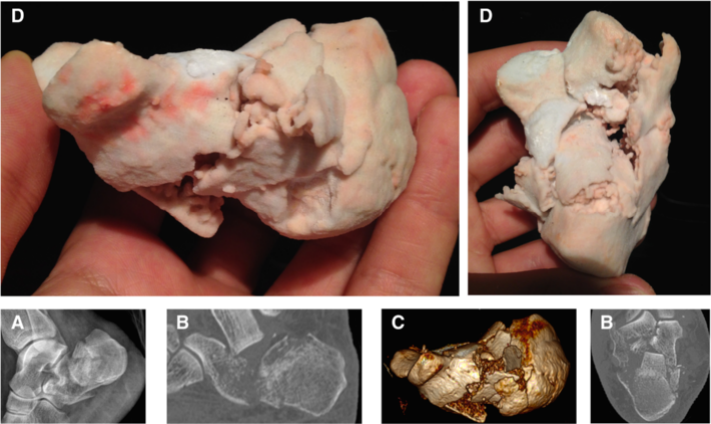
X ray (a), CT Scan (b), 3D Volume rendering (c) and 3D printed replica (d) of calcaneus fracture
